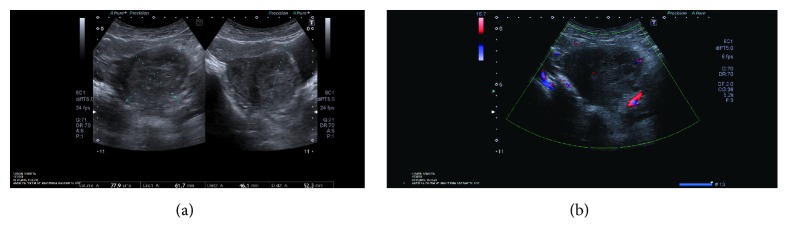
A repeat transvaginal ultrasound and color Doppler were done two months later; (a) the lesion was measuring 61 *∗* 46 *∗* 52 mm (77 cm^3^) and (b) revealed no blood flow.
